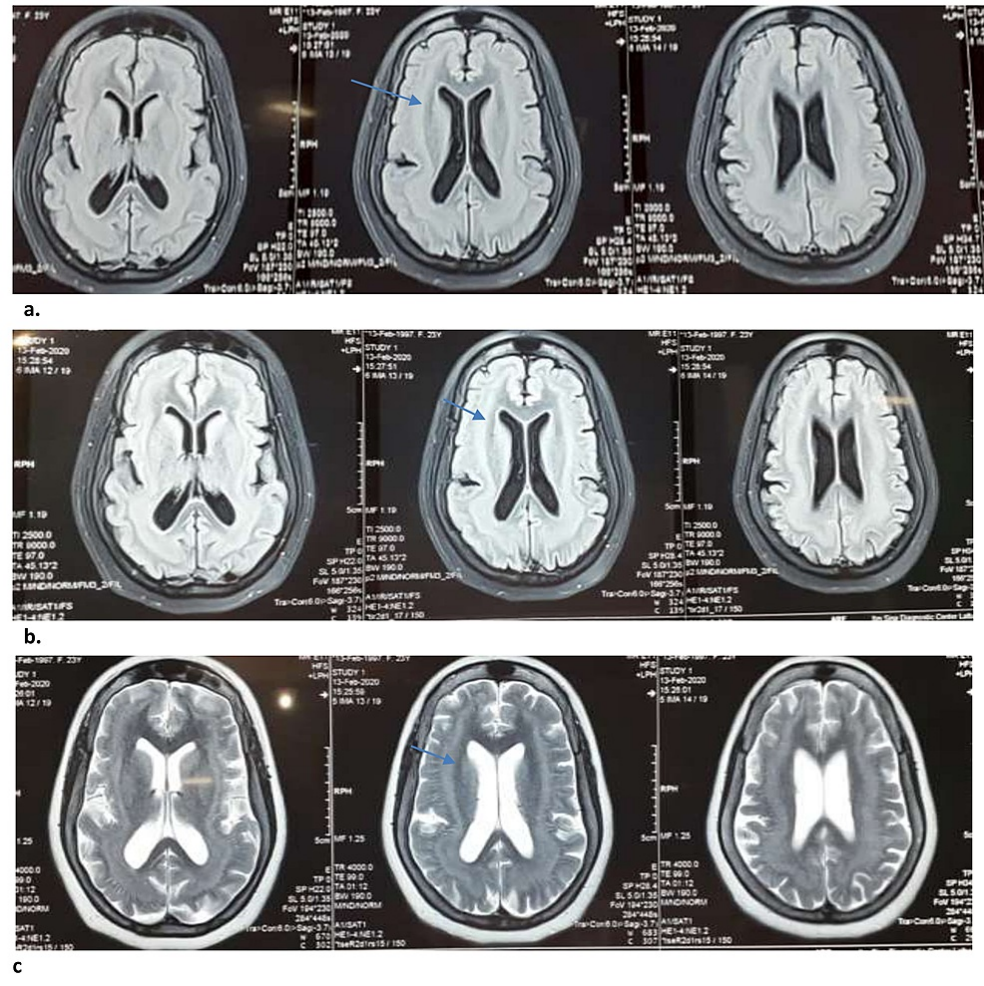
MRI of Brain T1 (top row, a ) T2 (middle row, b) FLAIR (bottom row, c) sequence revealed a complete band of gray matter located deep to, and roughly paralleling, pachygyric overlying the cortex. The thickness of the band was marked in posterior parietal and occipital region. FLAIR, fluid-attenuated inversion recovery
